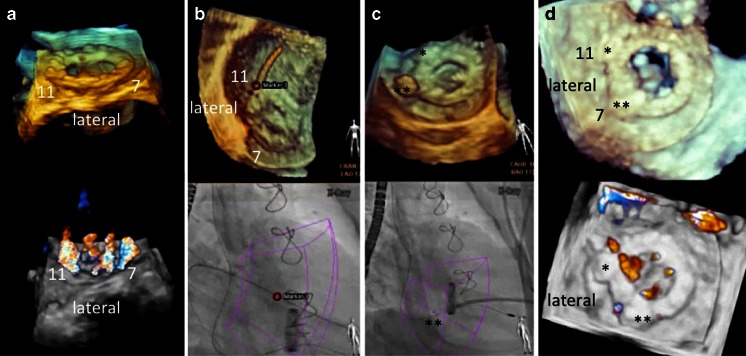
Fusion imaging during mitral paravalvular leak closure. 3D real-time echocardiography with and without color Doppler is helpful to determine the exact location and numbers of valvular and paravalvular jets (a). In this case, jets at 7 and 11 o’clock were considered the target lesions. b A *red marker* was used to locate the lesion at 11 o’clock (*upper image*), guiding the wire passage of the correct leak under fluoroscopy (*bottom image*). c The same approach was used to close the lesion at 7 o’clock with a vascular plug (*double asterisks*). d Echocardiographic images of the final result showing vascular plugs (*single* and *double asterisks*) at 11 and 7 o’clock, respectively, and a markedly reduced paravalvular and unchanged transvalvular regurgitation compared to a
